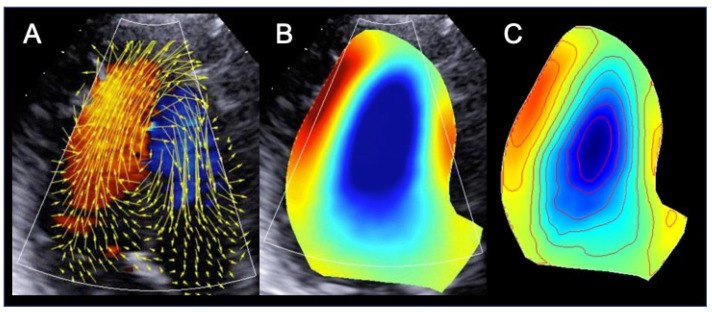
Intracardiac flow dynamics in a patient with dilated cardiomyopathy. Different maps were used to represent flow properties. The vector flow map (A) shows that the flow circulates along the posterolateral wall and is rotating anteriorly at the level of the left ventricular apex. In the circulation map (B) this translates into the formation of a single large vortex, that rotates clockwise (blue color) at the mid-apical portion of the left ventricle. The steady-streaming flow map of one heartbeat (C) shows the streamlines and the color map of the vorticity field. All images were obtained using the HyperDoppler software of an Esaote Mylab X8 echo-scanner without contrast injection.
